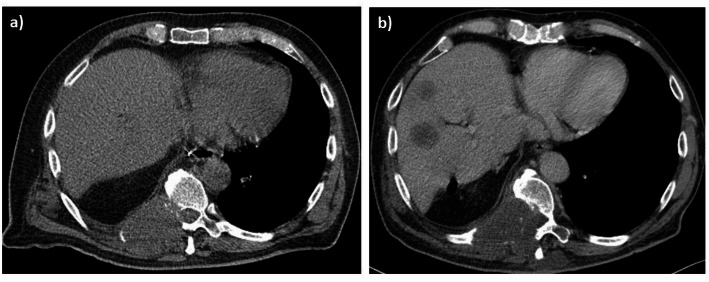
a Axial computed tomography (CT) imaging of a patient with locally advanced non-small lung cancer invading the spine before radiotherapy (RT) and b contrast-enhanced CT imaging with local and progressive disease after RT in the follow up with poor prognosis
